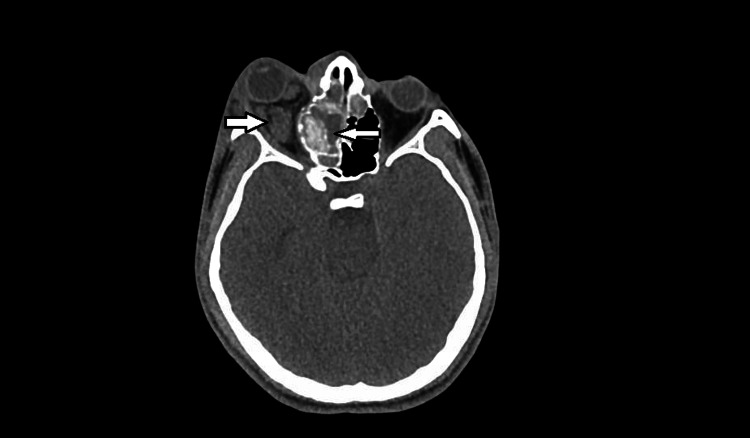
Initial CT Orbit showing sinusitis with orbital cellulitis and small fluid collection posterior to the eye without any globe deformity or ocular nerve compression.
